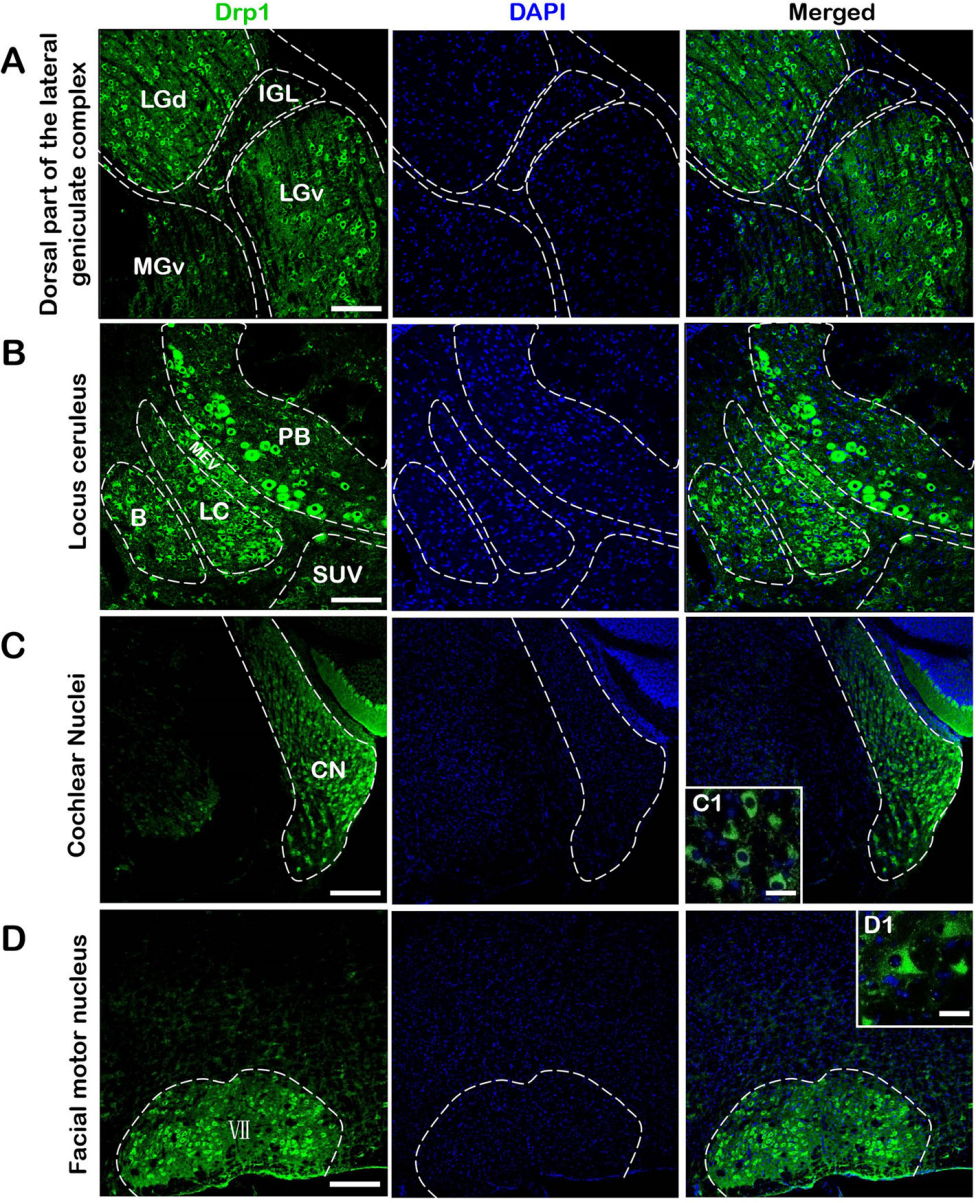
Brain regions with high Drp1 protein expression. Drp1 was highly expressed in LGd and LGv a, PB b, CN c and VII d. C1 and D1 show magnified images of C and D. Bar = 100 μm a, b; 200 μm c, d; 30 μm (C1, D1). Abbreviations: LGd: dorsal part of the lateral geniculate complex; LGv: ventral part of the lateral geniculate complex; IGL: intergeniculate leaflet of the lateral geniculate complex; MGv: medial geniculate complex, ventral part; PB: parabrachial nucleus; B: Barrington’s nucleus; LC: locus ceruleus; MEV: midbrain trigeminal nucleus; SUV: superior vestibular nucleus; CN: cochlear nuclei; VII: facial motor nucleus
